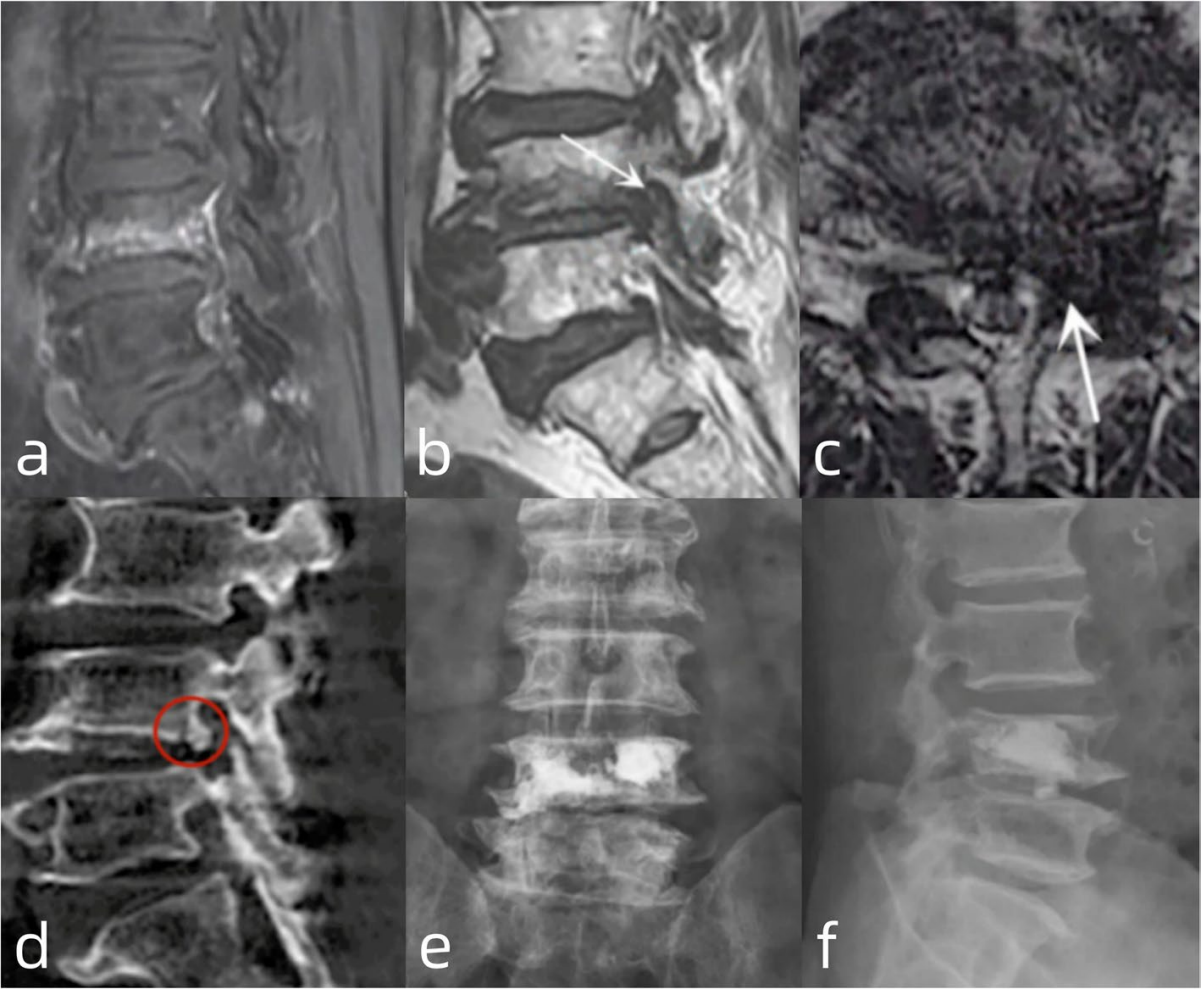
a Sagittal view of T2-weighted MRI showing fracture of L4 IEP; b, c: The sagittal and cross-sectional view of T2-weighted MRI identifying the stenosis of left-sided L4/5 foramen; d: Osteophyte and fractured fragment within the L4/5 foramen; e, f: The distribution of bone cement on anteroposterior and lateral view of postoperative radiography
